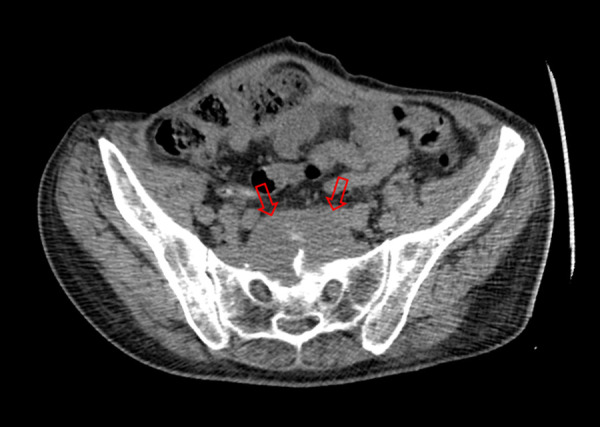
Transverse CT image showing deep pelvic abscess located at presacral region before drainage (red arrows).
